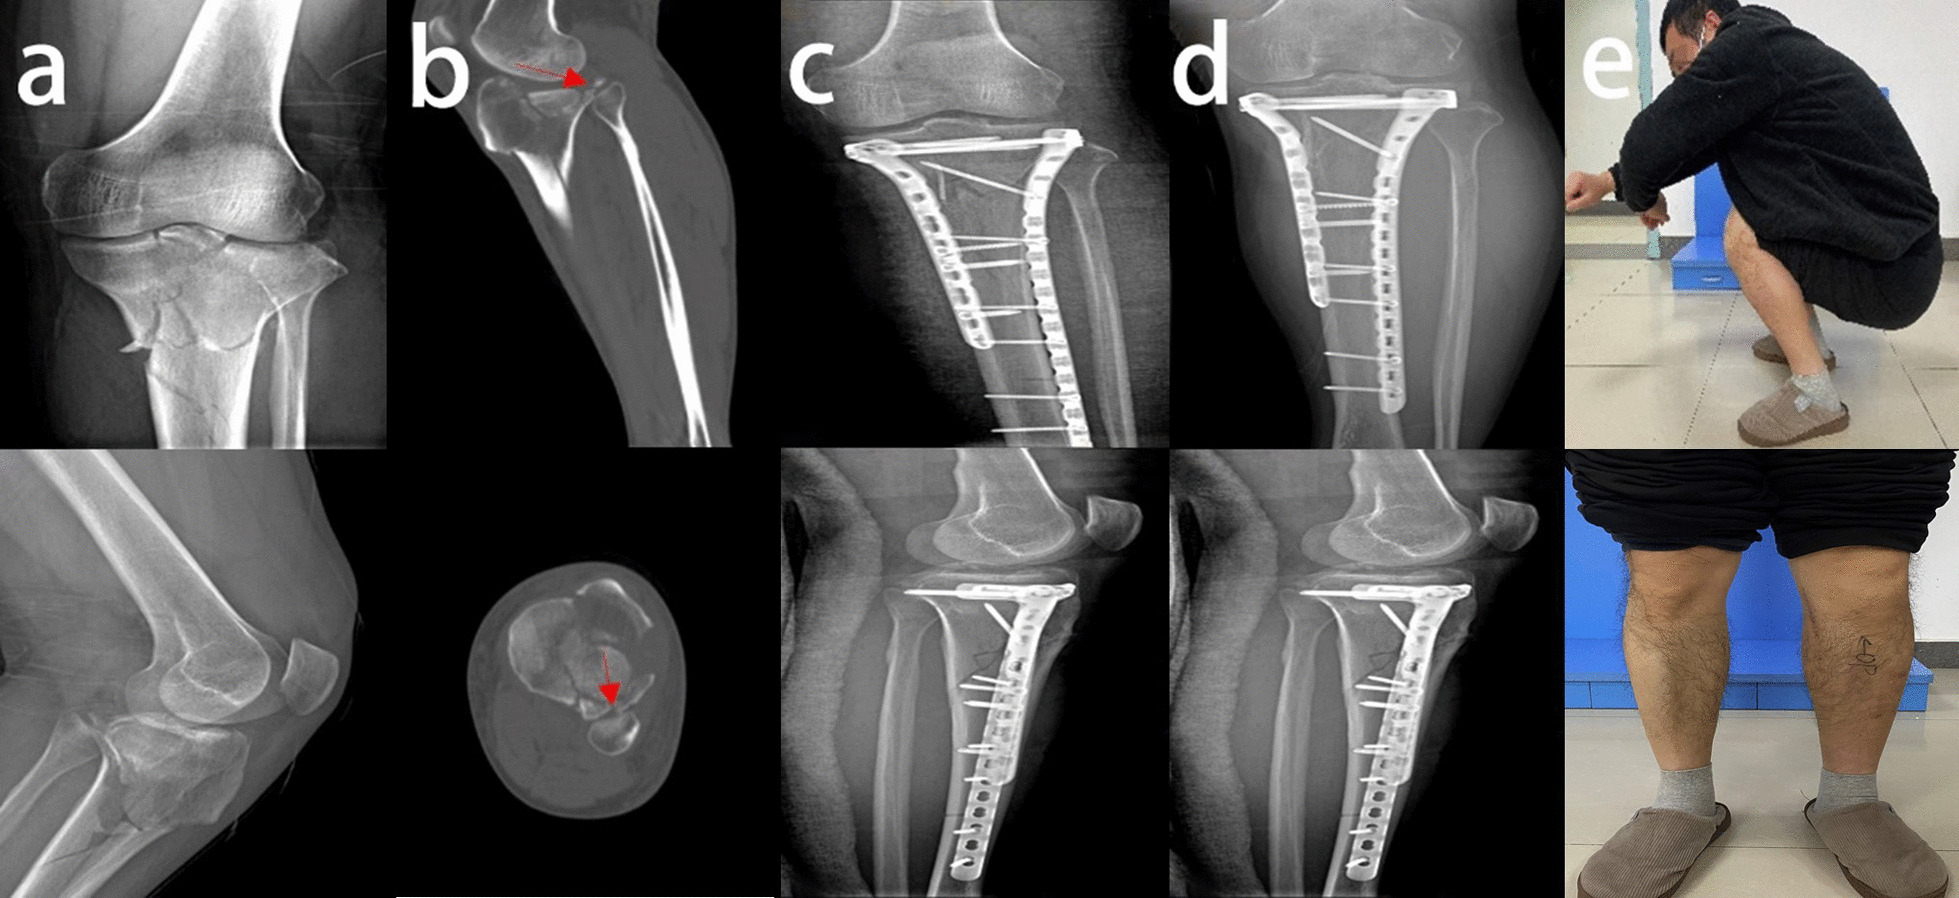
a Preoperative anterolateral radiographs of a 50-year-old male patient. b CT scan indicating PJF fracture (red arrow) with an intact proximal fibular. c Postoperative anterolateral radiographs. d Anteroposterior and lateral radiographs indicating good fracture healing four months after the operation. e Satisfactory knee motor function
